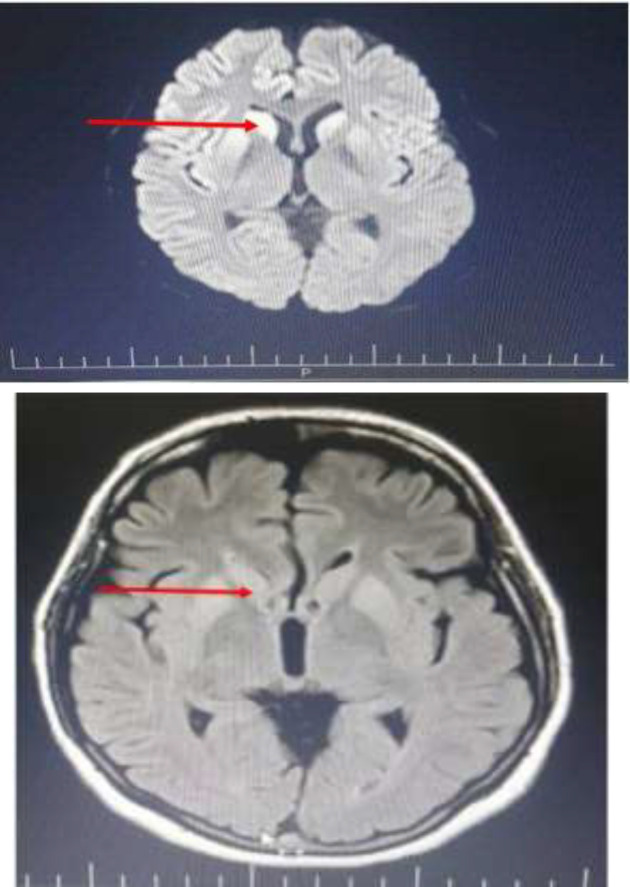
Brain MRI (Hyperintense signal in right and left head of caudate and putamen)
